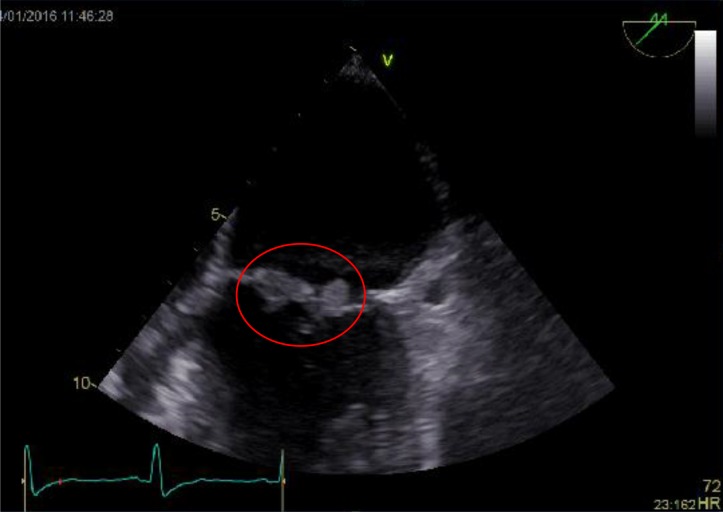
The mitral valve vegetation shown on a trans-thoracic echocardiograph (circled in red).
